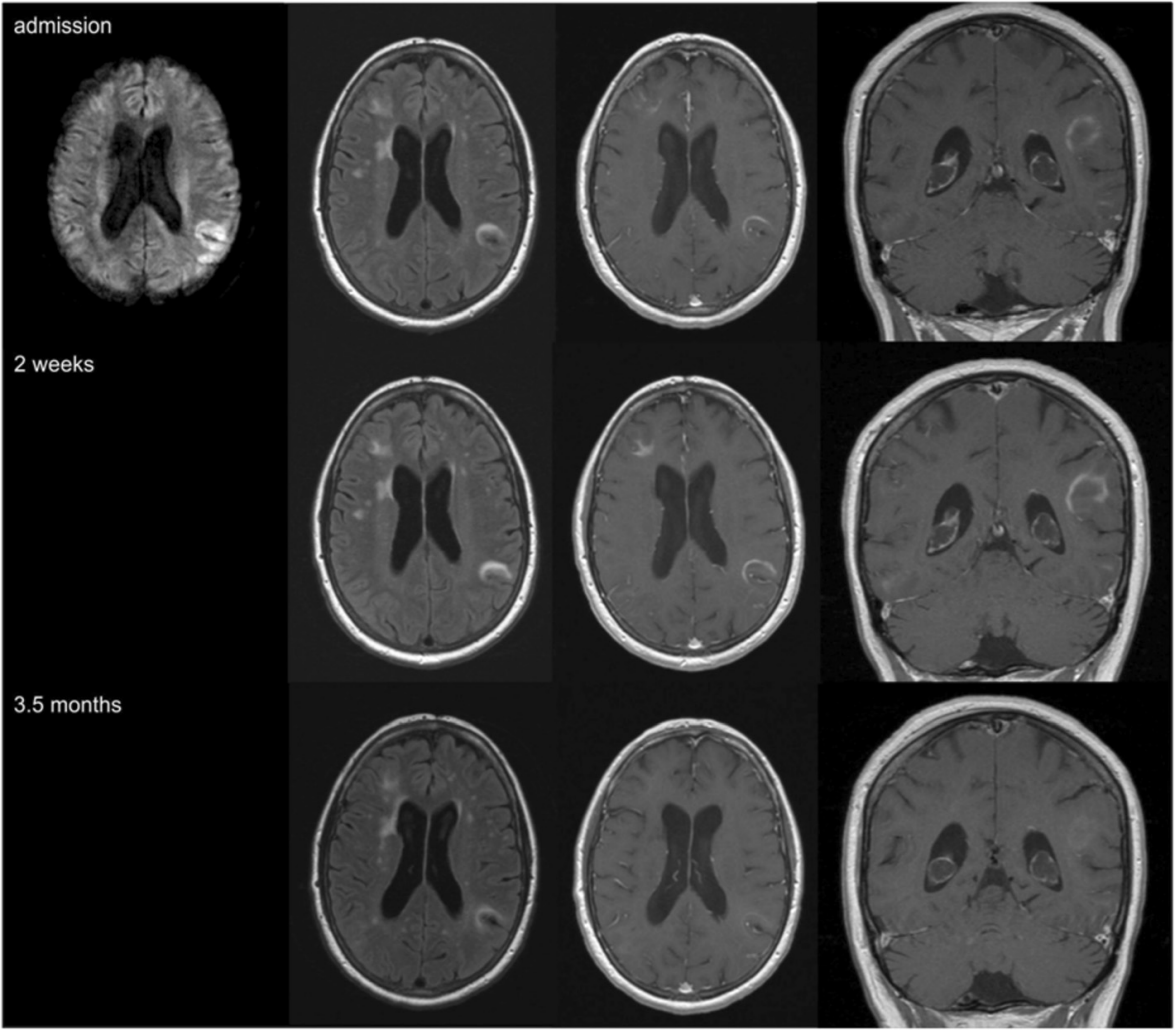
MRI of the patient’s brain in the course of the disease. shows MR imaging of the brain at the onset of symptoms and imaging controls after two weeks and 3.5 months. Several subcortical lesions are located in the right frontal and left parietal lobe and show progression during the first two weeks. They present hyperintense lesions in T2/FLAIR with partial increased signal in contrast-enhanced imaging.
